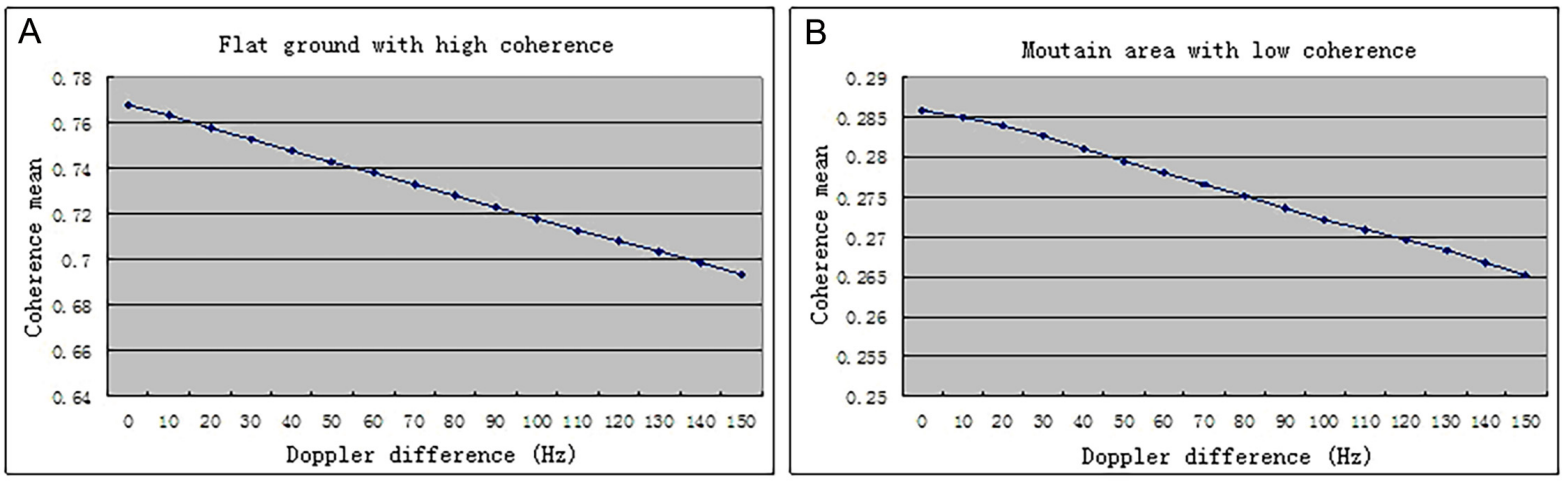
Doppler difference for flat ground and mountain area.
